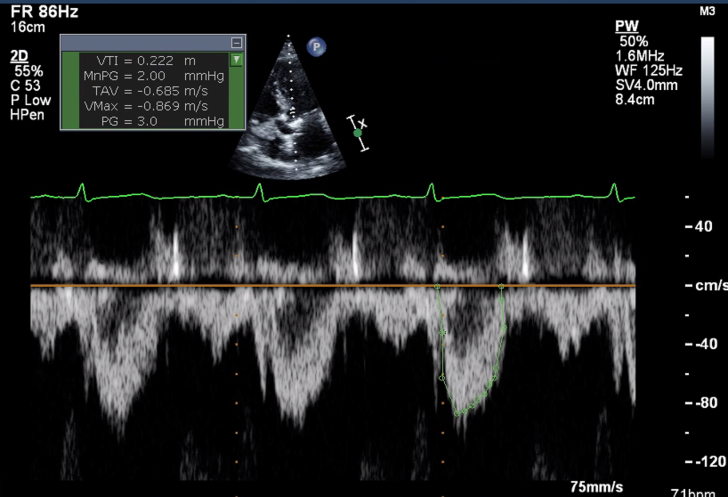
Apical 5-Chamber View Pulse Wave Doppler Assessment Apical 5 chamber: align PW sample volume in the left ventricular outflow tract avoiding the area of flow convergence.
